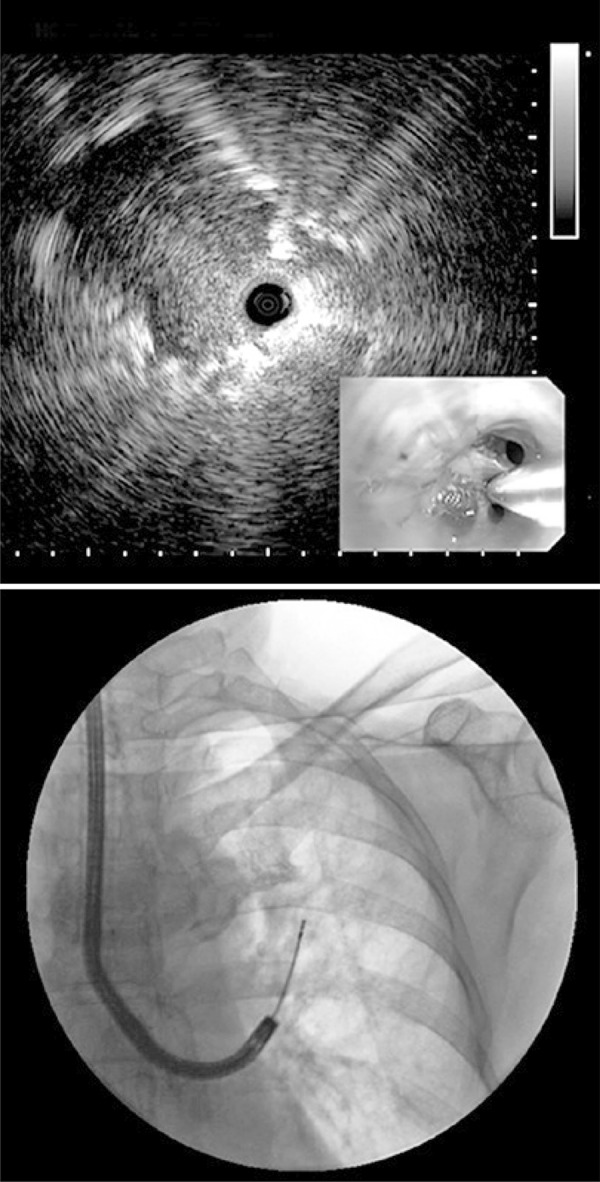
Radial endobronchial ultrasound and transbronchial biopsy guided by fluoroscopy
